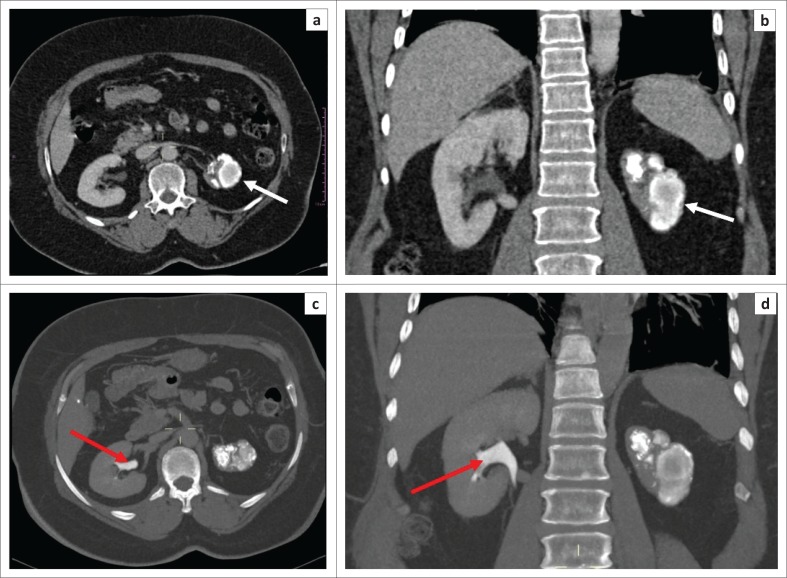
(a and b) Venous phase post-contrast computed tomography (CT) demonstrates a shrunken left kidney replaced by dystrophic calcifications (white arrow), in keeping with a ‘putty’ kidney. (c and d) Renal delay CT demonstrates contrast excretion in the right kidney (red arrow) and no contrast excretion in the non-functional left kidney, in keeping with autonephrectomy.
